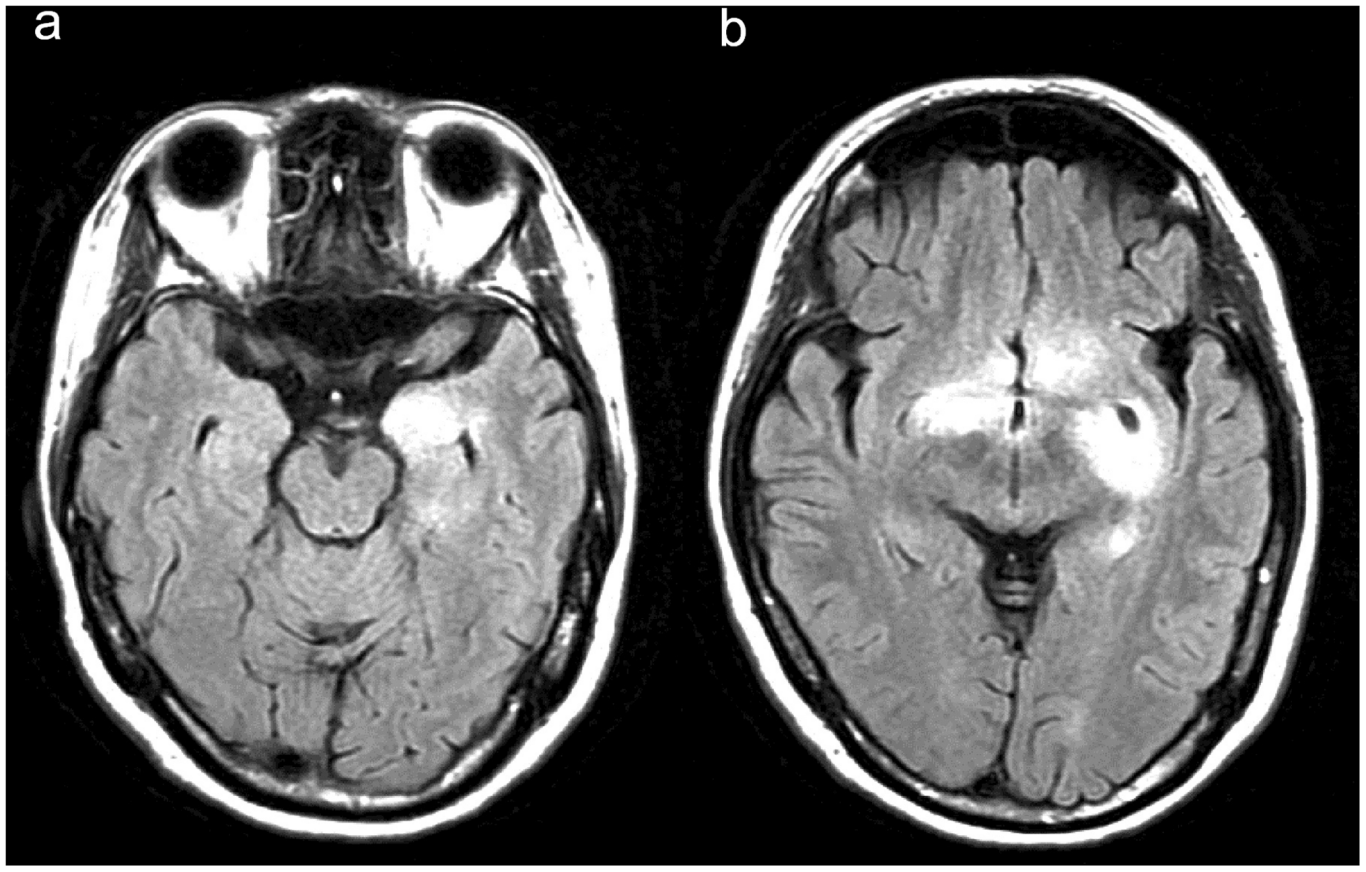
Fluid attenuated inversion recovery (FLAIR) magnetic resonance images (a, b) of another patient #7. They show multiple hyperintensity lesions over juxtacortical area of left medial temporal lobe and bilateral basal ganglion (caudate nucleus and putamen). Pons and cerebellum are also involved.
